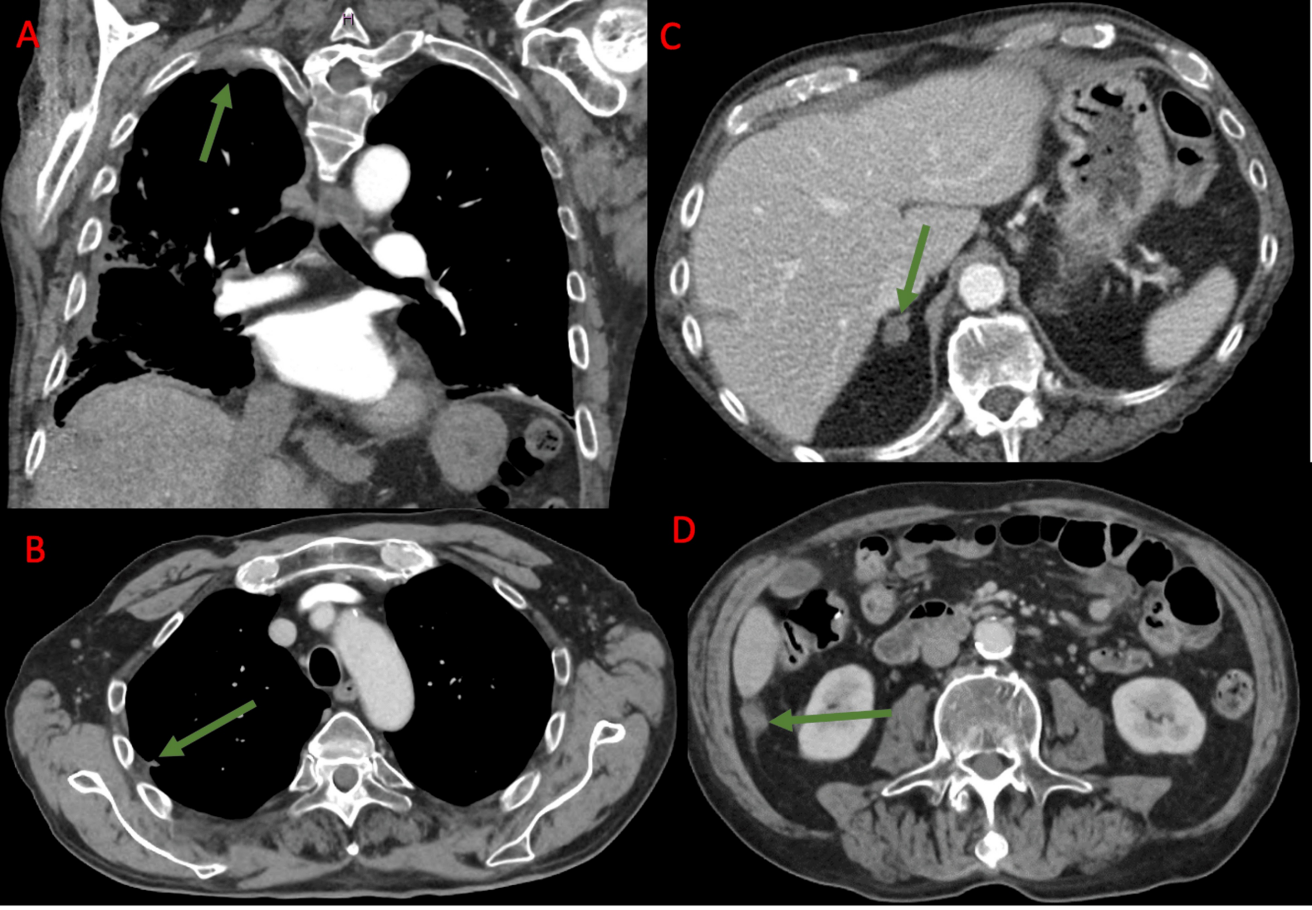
Target lesions post-ICI therapy demonstrated on computed tomography. (A) Right apical mass now measuring 3.4 cm. (B) Right pulmonary nodule now measuring 0.7 cm. (C) Adrenal nodule now measuring 1.2 cm. (D) R peritoneal nodule now measuring 1.3 cm. The green arrow points to the lesion of interest in each image. ICI: immune checkpoint inhibitors.
